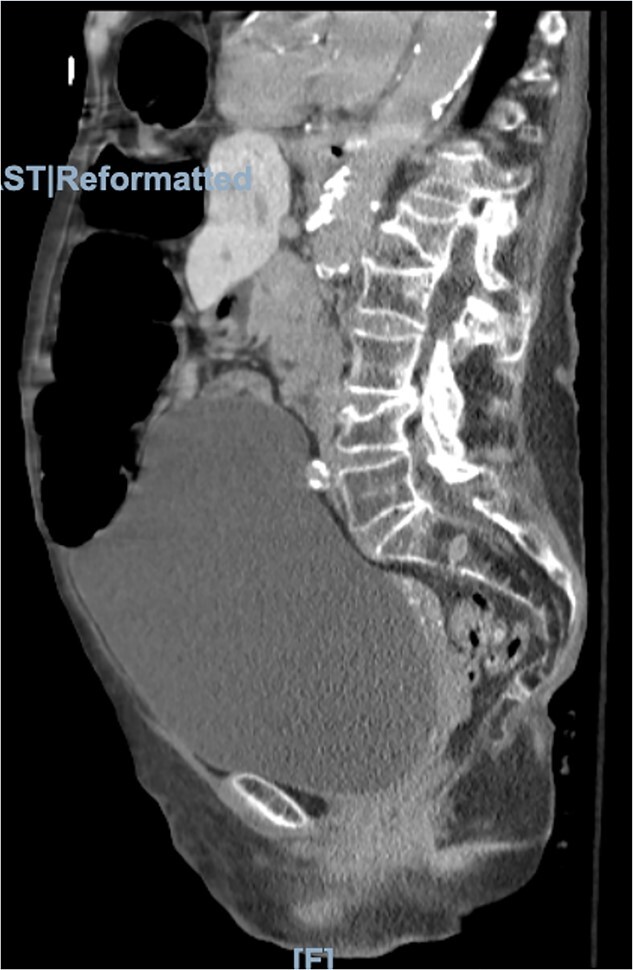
Morgagni hernia seen on CT abdomen and pelvis with contrast (sagittal view).
